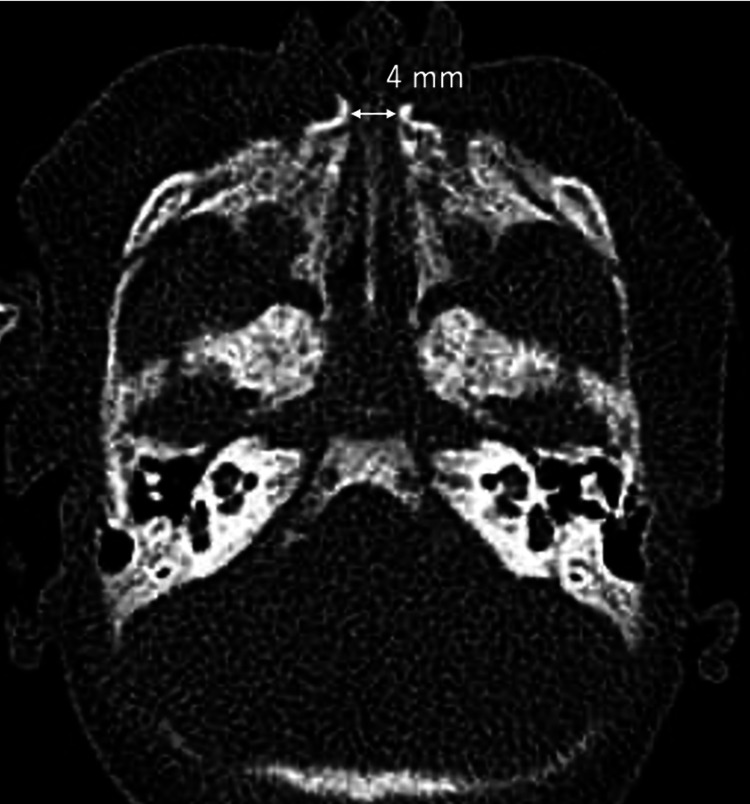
CT results on day 7 after birth. Axial CT scan on day 7 after birth showed a narrow nasal pyriform aperture. The width of the pyriform aperture was 4 mm.
